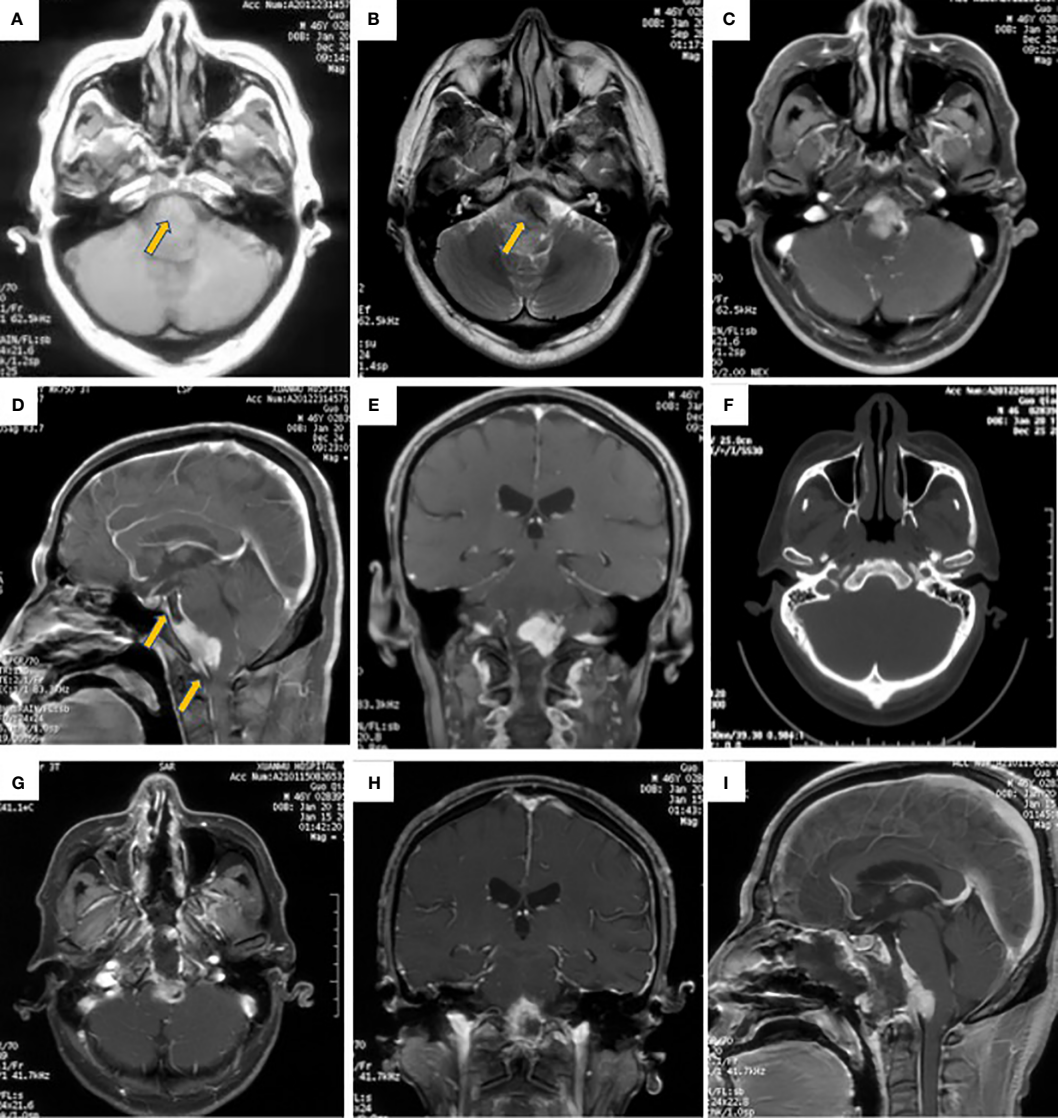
Brain MRI images showed intracranial mass located at middle of upper clivus region. (A, B). The lesion located at middle of upper clivus region presented with isointense signal in T1-weighted MRI image (A, the arrow), and hypointense signal in T2-weighted MRI image (B, the arrow); (C, E): The mass was uniformly enhanced after contrast MRI scan, and the base of the tumor was extensively stick to the adjacent dura and brain stem, showing meningeal tail sign (the arrow in D), and meningioma was highly suspected. (F). CT bone window showed no bone invasion. (G, I). The MRI showed partial resection of the lesion.
